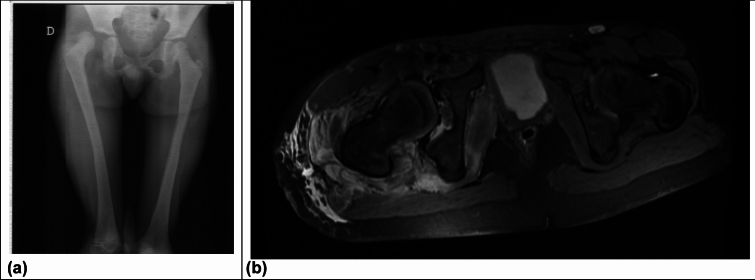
Patient 2 a After accident, X-ray pelvic overview, posterior dislocation; b Five days after accident, MRI with soft tissue interpositions
